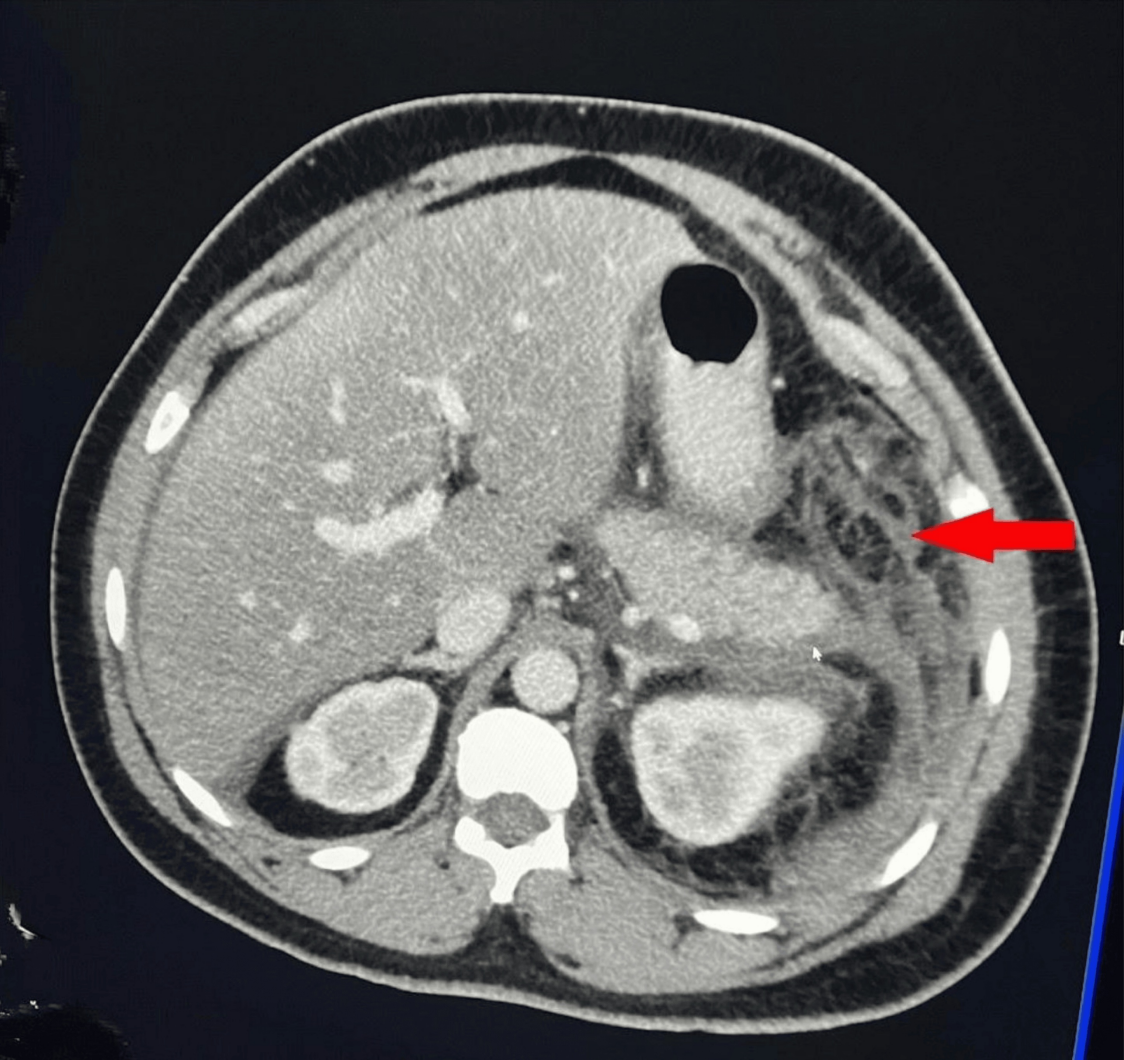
Axial view of the CT scan demonstrates extensive pancreatic inflammation and peripancreatic fat stranding consistent with acute pancreatitis (indicated by the red arrow) CT: Computed tomography
